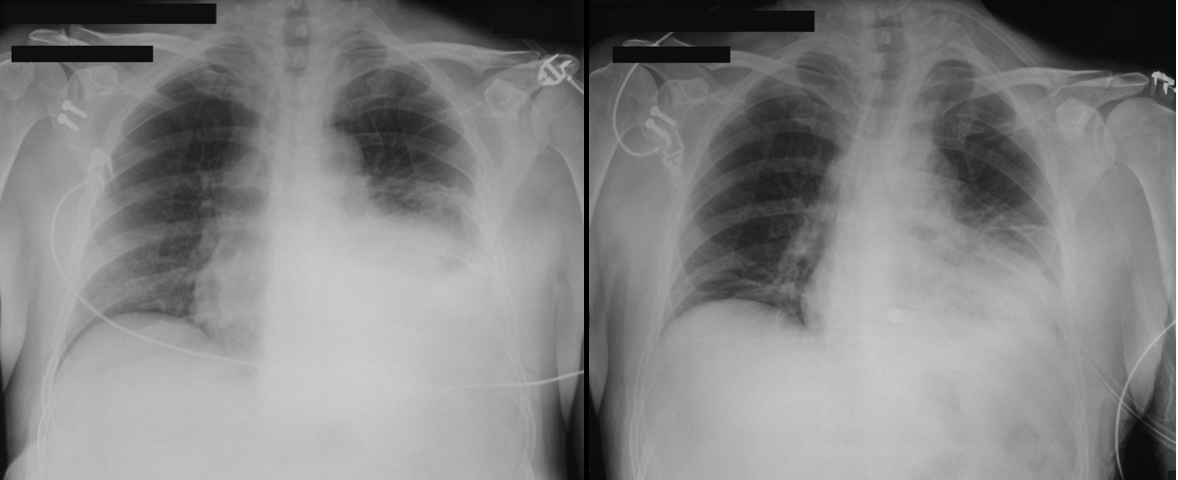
Chest radiograph showing a) left hemothorax and enlarged cardiac silhouette b) residual hemothorax and persistent enlarged cardiac silhouette after pleural tube drainage.
